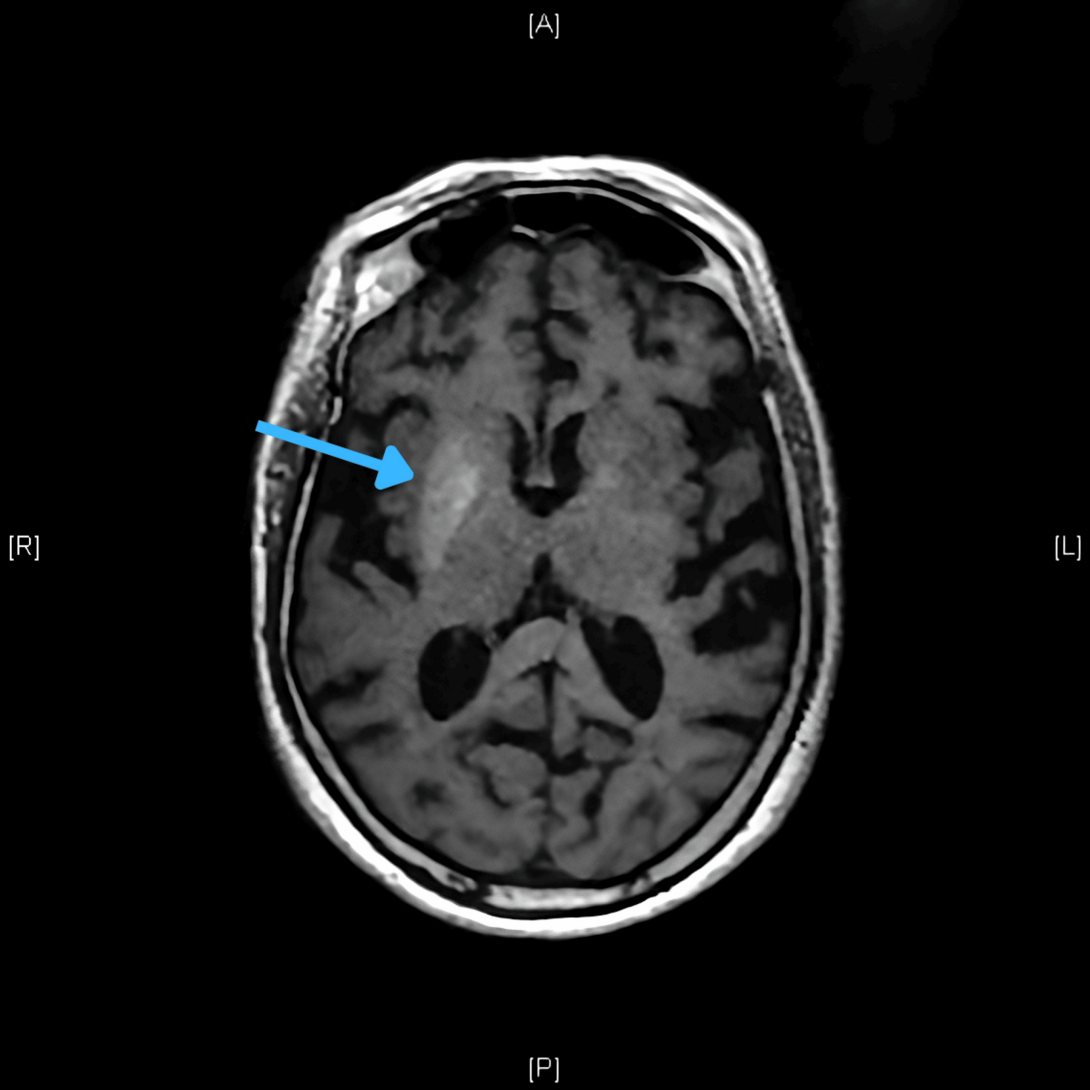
MRI with an axial view of T1 weighting highlighting spontaneous hypersignal in the affected basal ganglia (contralateral to the choreiform limb).
